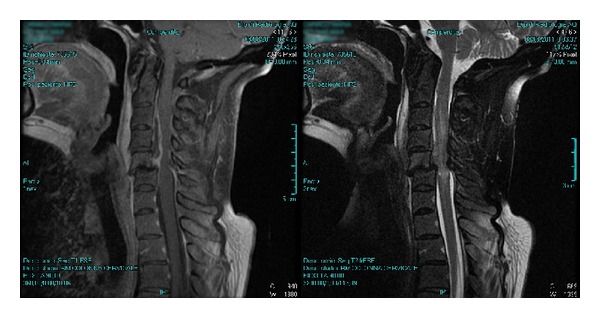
MRI Case  1: altered signal intensity with synovial effusion in interapophyseal joints C5-C6 and C4-C5, associated with extensive alteration of signal paravertebral soft tissues on the left paravertebral front and retropharyngeal from C1 to C7; there is also extensive alteration signal in correspondence with the epidural space anterior and lateral left from C3 to C6 and more evident in C4-C5 left side. Segmental stenosis of the spinal canal at C5-C6, with compression on the spinal cord and the abolition of the subarachnoid space perimedullary, is evident. The spinal cord has altered signal intensity level from C3 to C6. Framework compatible with the involvement of nature-infectious inflammatory type septic arthritis interapophyseal with paravertebral and epidural abscesses and myelopathy.
